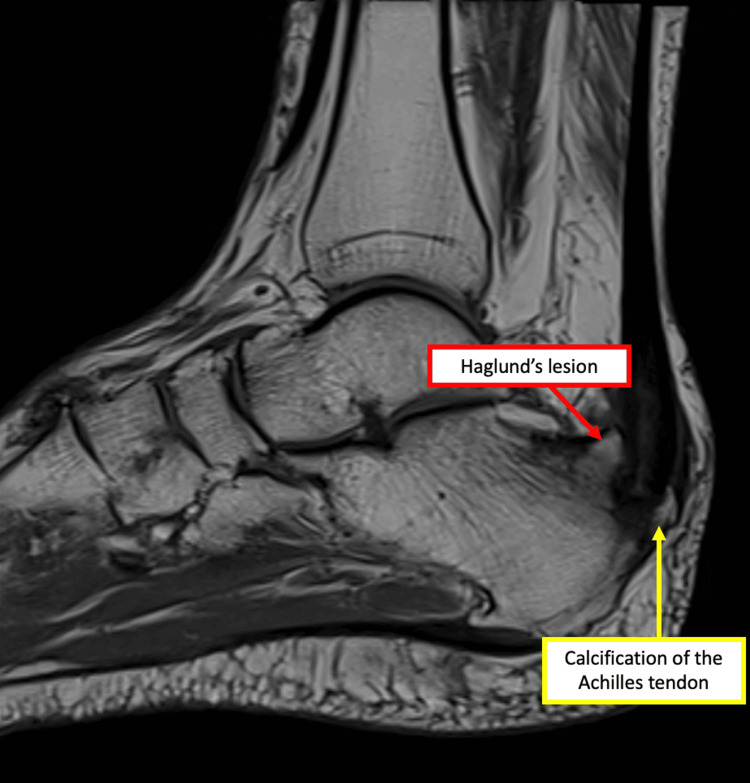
Calcaneal prominence and Achilles tendon insertion changes in Haglund syndrome on MRI Sagittal T1-weighted MRI sequence of the right ankle demonstrating prominent posterosuperior calcaneal tuberosity (Haglund deformity) associated with calcification of the Achilles tendon distal insertion. MRI: magnetic resonance imaging
